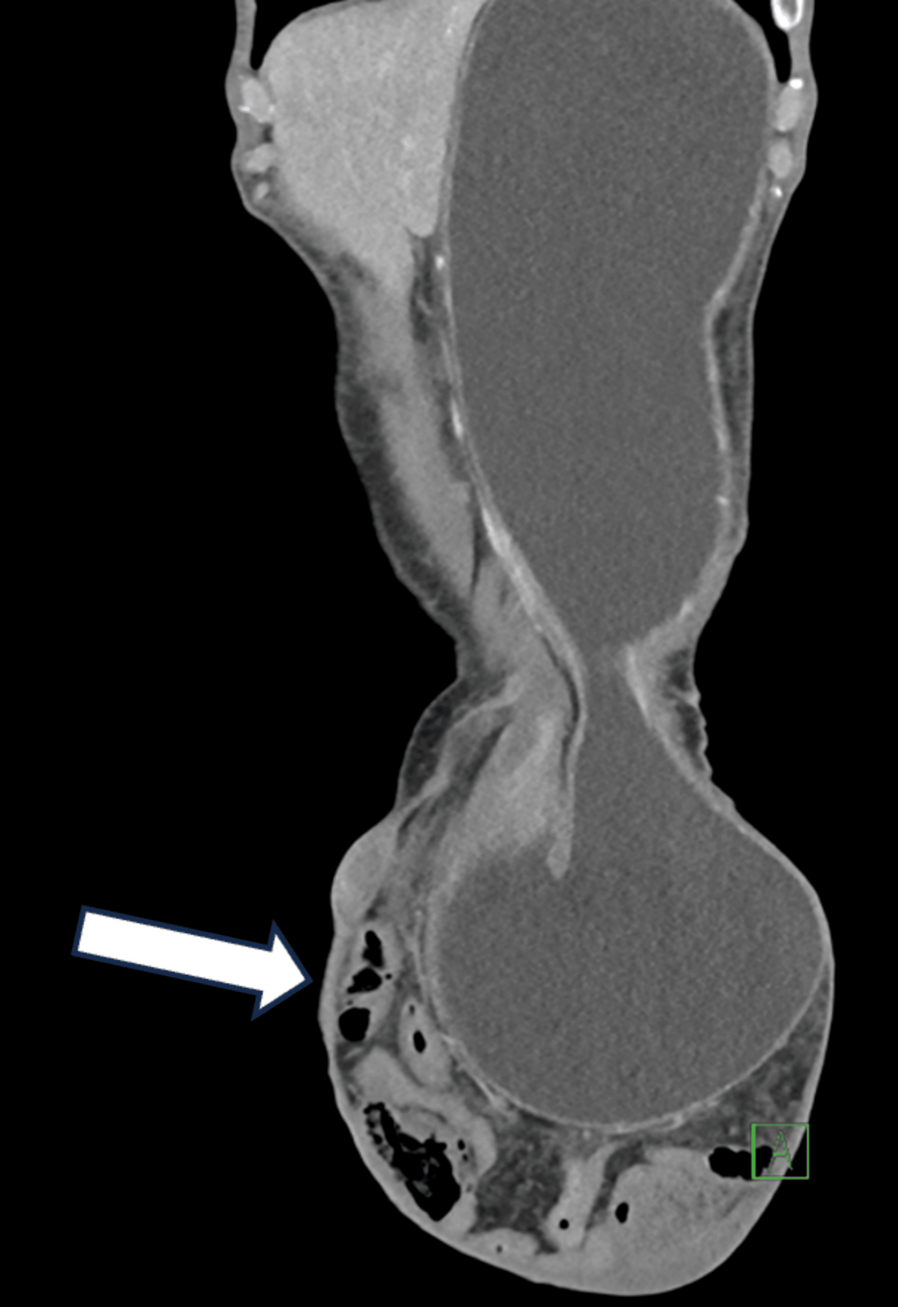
Computed tomography of the abdomen and pelvis coronal cross-section showing dilated stomach extending into the left inguinal hernia.
